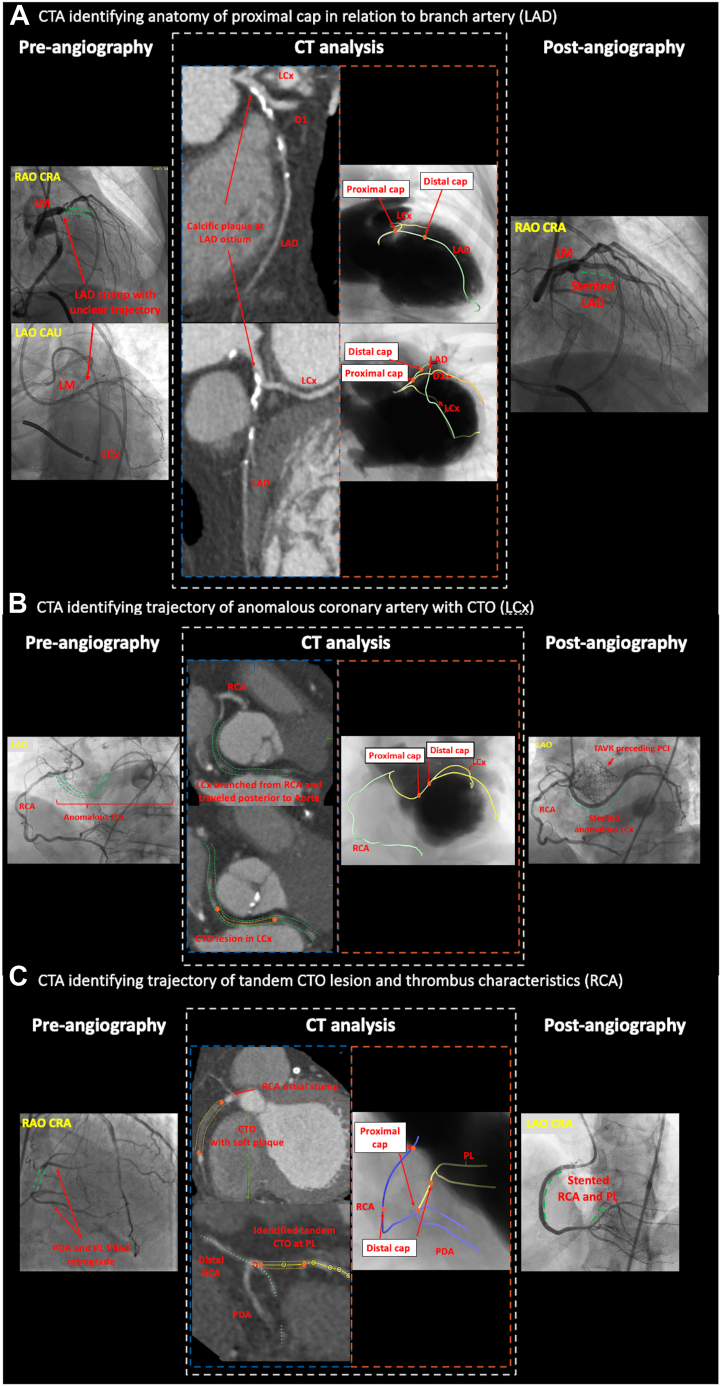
CT pre-planning of the cases and angiographic outcomes. (A) Diagnostic angiography showed LAD stump with unclear trajectory (Supplemental Video 1). CCTA-curved MPR images identified proximal cap morphology and ostial LAD take-off in relation to D1 and LCx. Coregistered roadmap created to delineate ambiguous LAD CTO take-off. LAD trajectory is delineated as green, LCx as yellow, and D1 as orange. Interrupted green line represents CTO lesion in the LAD before and after stenting. (B) Diagnostic angiography showed retrograde filling of anomalous LCx CTO originating from RCA (Supplemental Video 2). CCTA MPR images identified fibrotic morphology of the proximal/distal cap and retroaortic course of the anomalous LCx. Coregistered roadmap visualized the length of occlusion. RCA trajectory is delineated as green and anomalous LCx as yellow. Interrupted green line represents trajectory of anomalous LCx before and after stenting. Interrupted orange line represents the CTO lesion. (C) Diagnostic angiography showed RCA ostial CTO with unclear trajectory and retrograde filling of PDA and PL (Supplemental Video 3). CCTA MPR images identified fibrotic morphology of proximal/distal caps and tandem CTO lesions. Coregistered roadmap created to delineate tandem lesion and guide angle of approach. RCA and PDA trajectory are delineated as blue and PL as yellow. Interrupted green line represents trajectory of RCA and PDA before and after stenting. Interrupted orange line represents the CTO lesions. CCTA, computed coronary tomographic angiography; CTO, chronic total occlusion; D1, first diagonal; LAD, left anterior descending; LCx, left circumflex; MPR, multiplanar reformation; PCI, percutaneous coronary intervention, PDA, posterior descending artery, PL, posterolateral, RCA, right coronary artery, TAVR, transcatheter aortic valve replacement.
